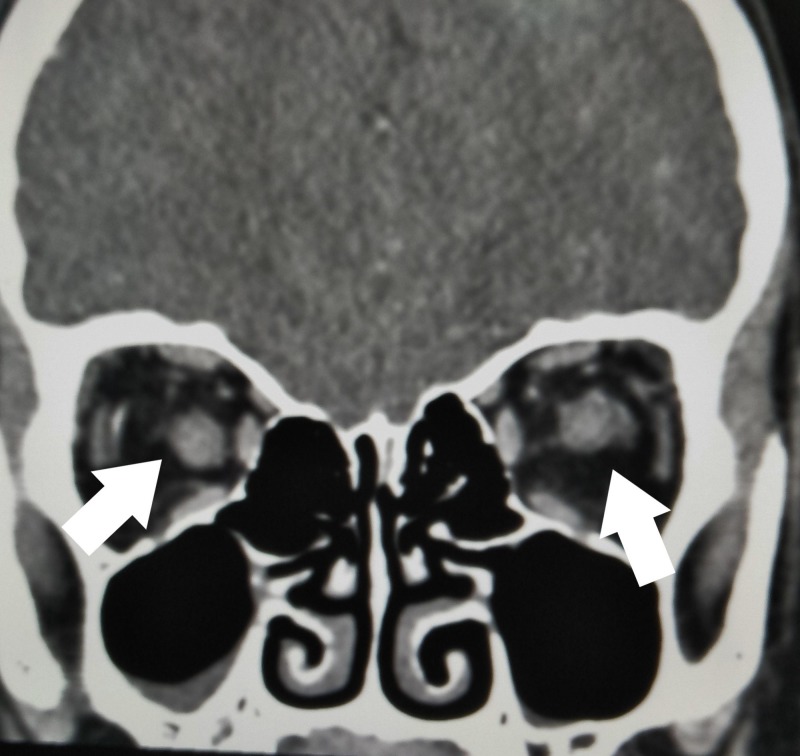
Computed tomography (CT) of the optic nerves Coronal view showing the left optic nerve is larger than right optic nerve (arrows)
